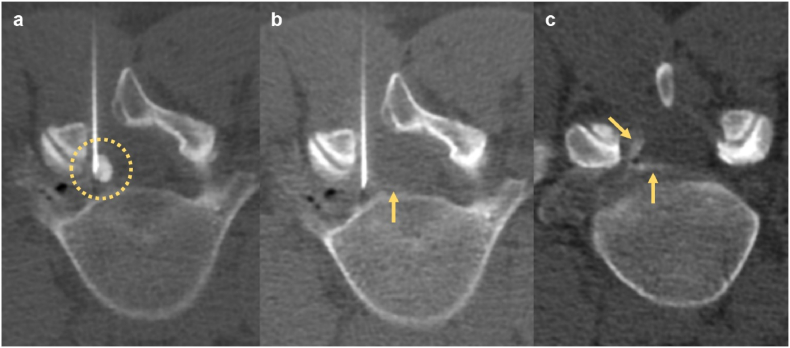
CT-guided discal cyst aspiration, fenestration and rupture in a 30 year old female with a post-operative left L4-5 discal cyst following prior same-level discectomy. (a) Axial intra-procedural CT demonstrates percutaneous needle placement within a left paracentral discal cyst, opacified with contrast (open circle). (b) Axial intra-procedural CT following initial cyst aspiration confirms absence of residual contrast in the cyst. Note the absence of contrast in the ventral epidural space (arrow). (c) Axial post-procedure CT following fenestration and rupture of the discal cyst and epidural steroid injection demonstrates restoration of normal epidural contours, opacified with contrast (arrows). This confirms complete resolution of the discal cyst.
